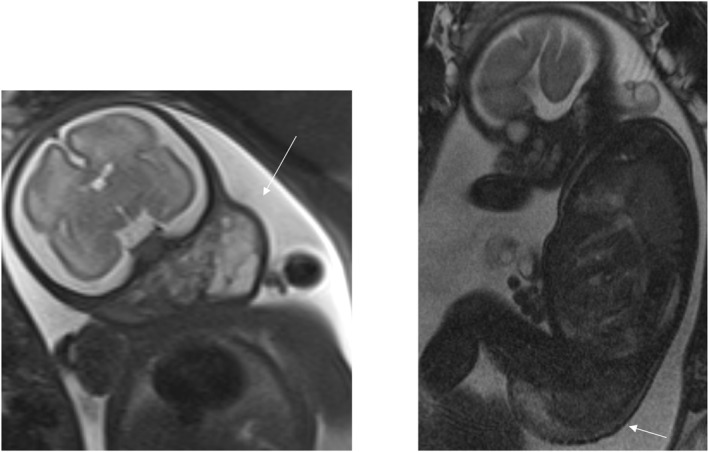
Fetal MRI images of Kaposiform lymphangiomatosis. Left image ‐ T2 haste coronal image of 27 weeks fetus with a multiloculated fluid filled cystic lesion that extends from the subcutaneous tissues on the left side of the neck anteriorly and posteriorly toward the midline. Extends from shoulder to ear with possible areas of hemorrhage. Right image ‐ T2 sagittal image of the same fetus showing a similar cystic lesion over the left buttock and thigh.
